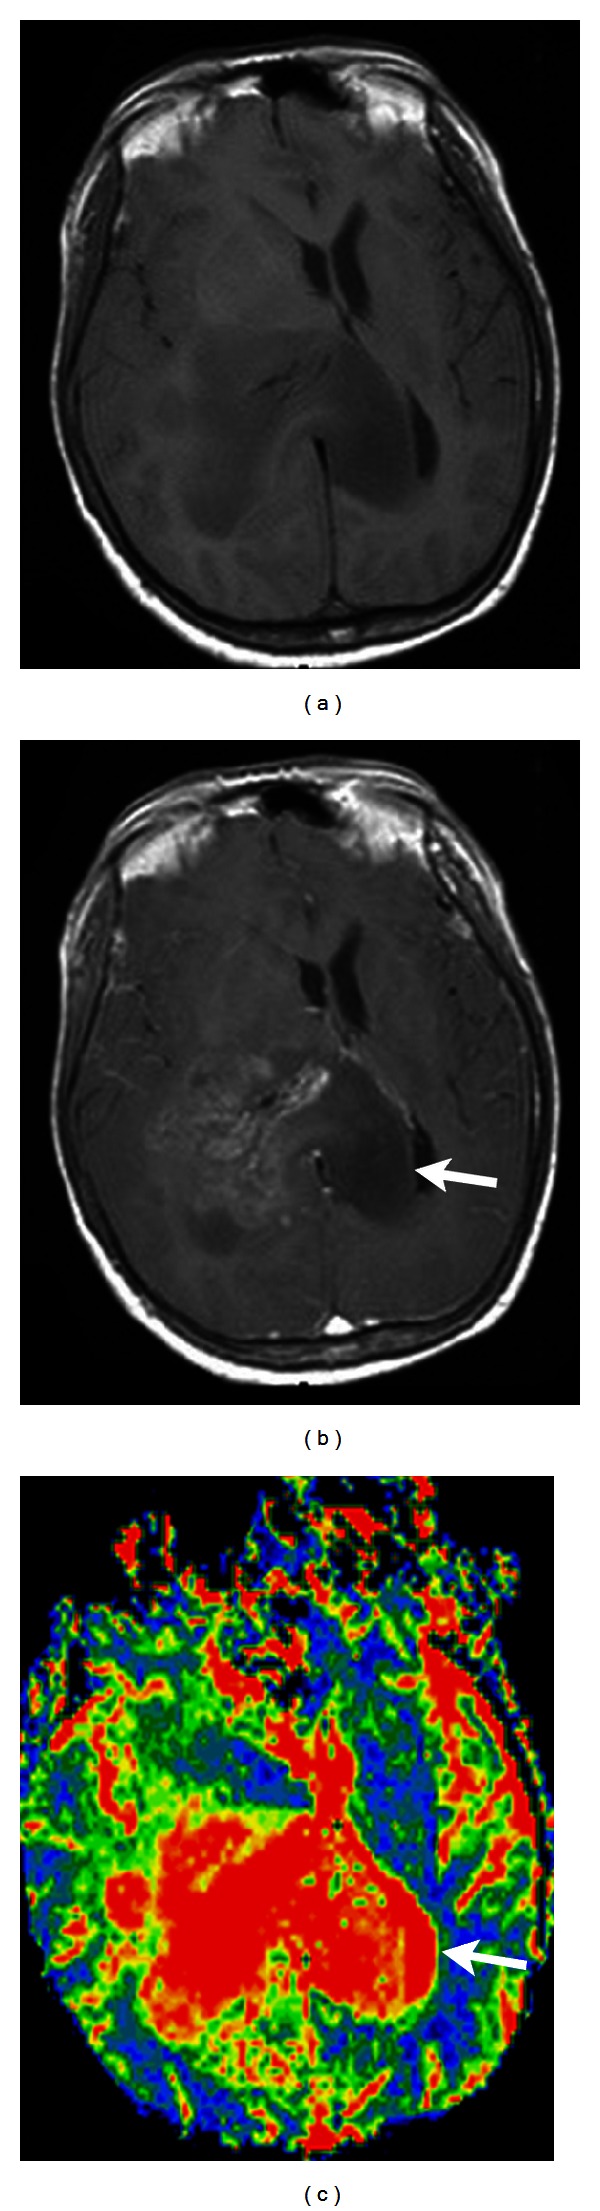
(a) Contrast-enhanced T1-weighted image shows a butterfly glioblastoma, involving the genu of corpus callosum. (b) The right aspect of the lesion appears to be heterogeneously contrast enhanced. (c) On rCBV map, the nonenhanced left aspect of the tumor (arrow) shows high rCBV (arrow in (c)). This helps differentiate tumor infiltration from perifocal edema.
